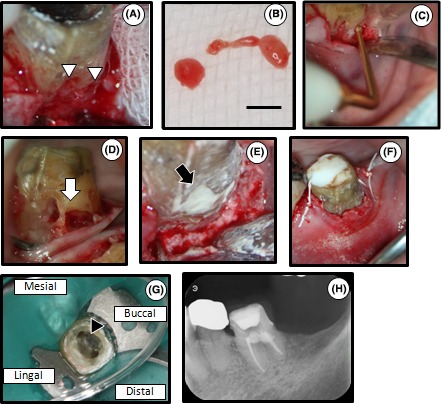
The first stage of treatment: removal of the granulomatous tissue, endodontic treatment and MTA filling of the defect. A, The surgical site. The cervical resorptive site was housed with the granulomatous tissue (white arrowheads). B, A part of the granulomatous tissue removed by the spoon excavator. Scale bar=3 mm. C, Complete removal of the granulomatous tissue by sonic instrument. D, Intraoral photograph after the removal of the granulomatous tissue. Pulp exposure measuring approximately 3 mm in diameter was observed at the resorptive site (white arrow). E, Filling of the resorptive site with MTA (black arrow). F, Tooth 36 after suturing. G, Location of root canal orifices and hardened MTA. Hardened MTA was observed (black arrowhead). The patient exhibited no clinical symptoms. H, Dental radiograph after root canal filling
